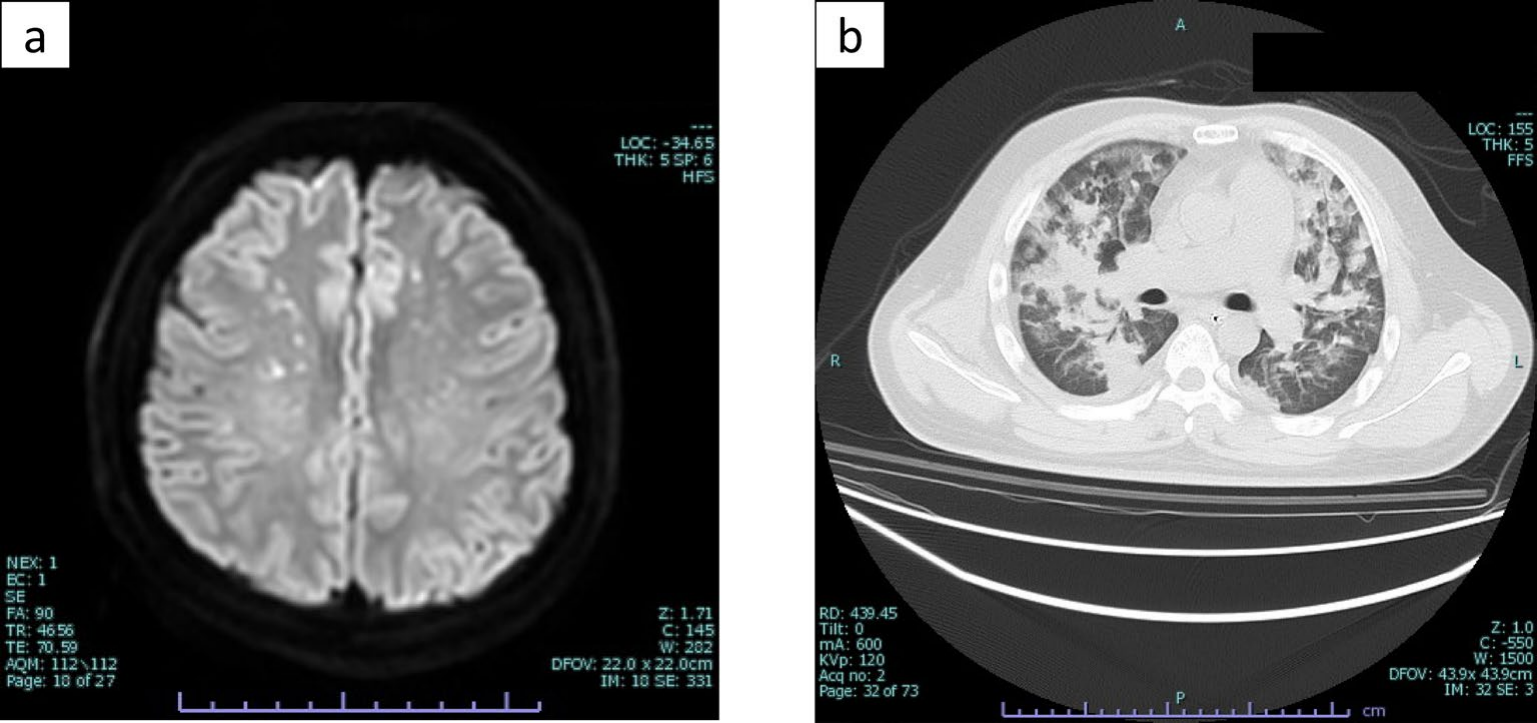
Perioperative images during the course of treatment. (a) Head MRI on Day 5 shows “starfield pattern”; (b) Chest CT on Day 5 shows signs of acute respiratory distress syndrome. CT, computed tomography; MRI, magnetic resonance imaging.
